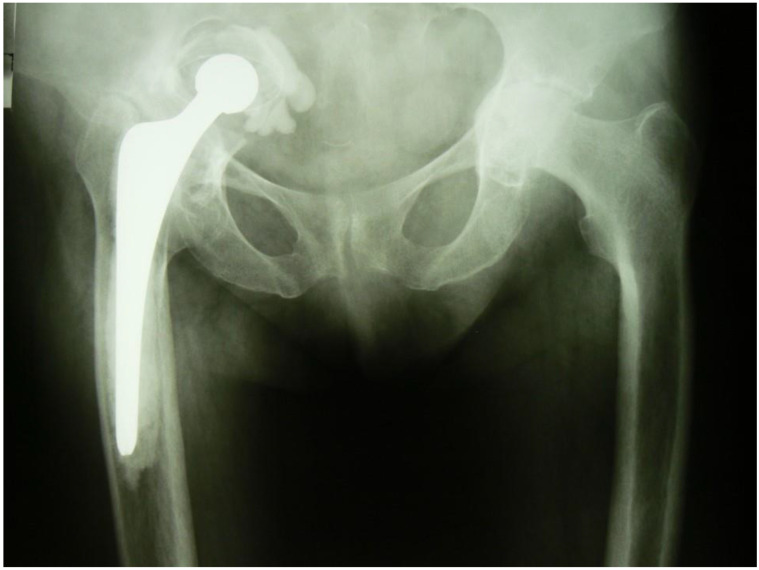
Protrusion of the prosthetic acetabulum, migration of the acetabular component, head and proximal part of the stem above the iliopectineal line. An antero-posterior (AP) radiograph of the pelvis and the hip joints.
